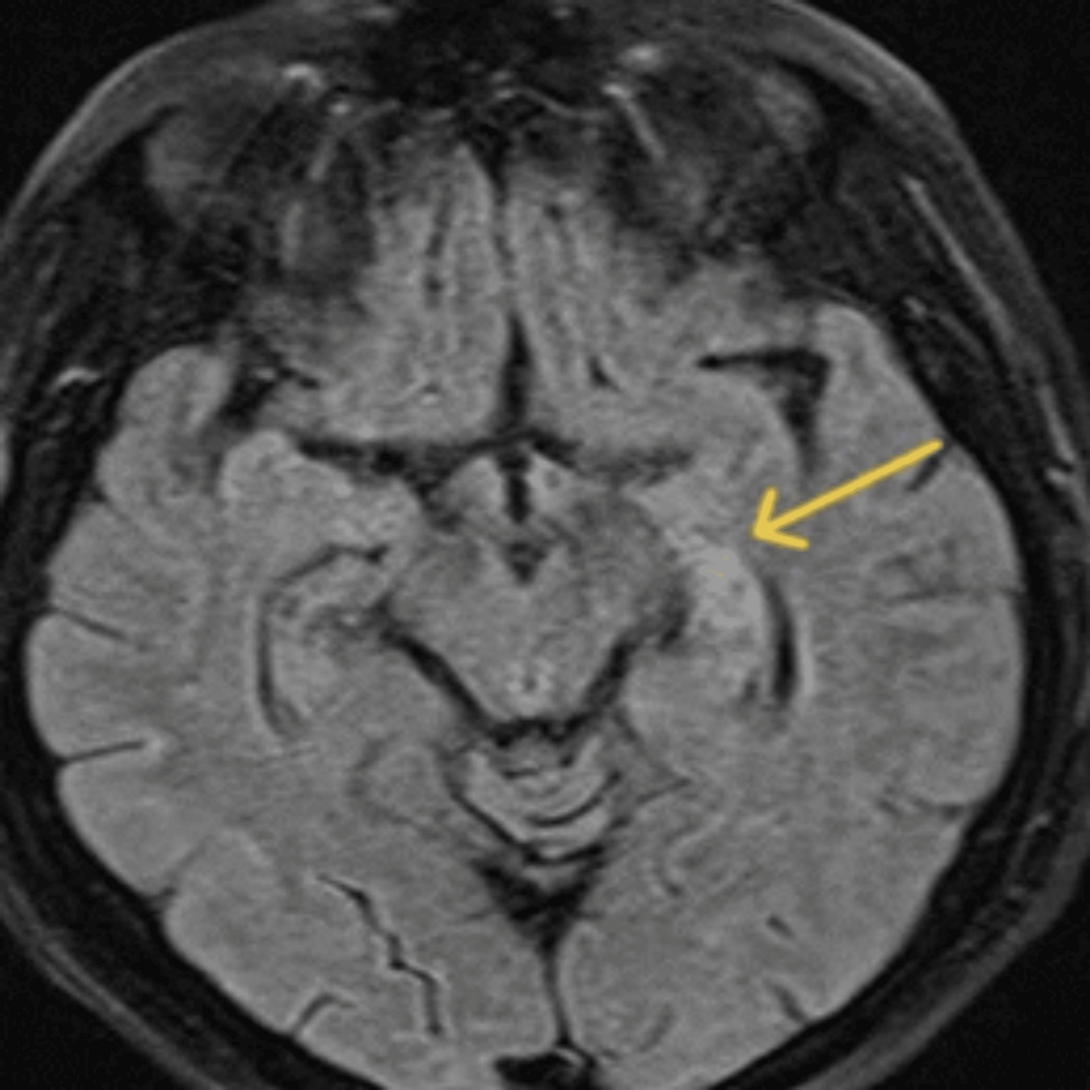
Axial T2-weighted FLAIR brain MRI The arrow shows a high signal area at the body of the left hippocampus. FLAIR - fluid-attenuated inversion recovery
